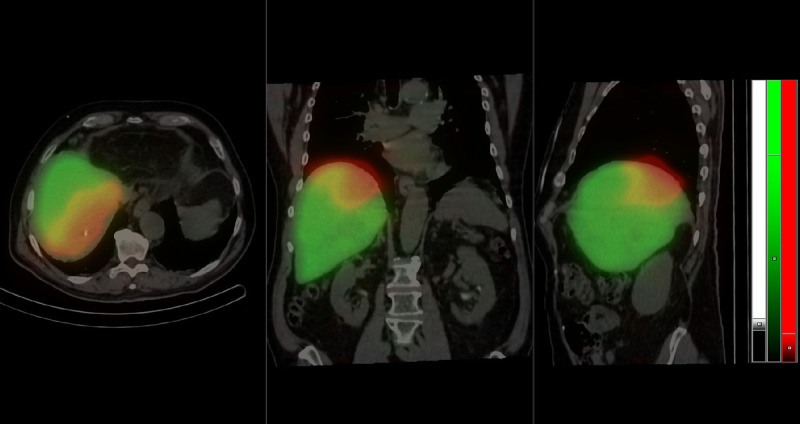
Fused ^99m^Tc-mebrofenin (green) and ^166^Ho (red) dual-isotope SPECT and CT images, transversal left panel, coronal middle panel, and sagittal right panel. Images were acquired on a Mediso SCP SPECT/PET/CT triple-modality camera (Mediso Medical Imaging Systems Ltd) equipped with a triple-detector SPECT after the transarterial injection of 140 MBq ^166^Ho-microspheres (QuiremScout; Quirem Medical B.V.) in the angiographic laboratory and the subsequent IV administration of 270 MBq of ^99m^Tc-mebrofenin. The healthy liver tissue included in the potential irradiation target of the planned radioembolization is marked by the yellow penumbra on the SPECT/CT images. The majority of the liver, however, expresses only ^99m^Tc-mebrofenin uptake (green), and the target encompassing the tumor is covered by the deposition of ^166^Ho-microspheres (red). Volumes of interests were drawn around the respective isotope distributions, and calculations from the encompassed volumes showed an estimated functional liver remnant (%FRL-C) of 85.5%, which was critically important considering that the patient had previously undergone a left hemihepatectomy. Fifteen days after the scout imaging, the radioembolization treatment was performed with 5 GBq of ^166^Ho-microspheres (QuiremSpheres; Quirem Medical B.V.) injected transarterially from the same catheter position. Posttreatment ^166^Ho-SPECT/CT images showed an identical microsphere deposition to that of the scout image set, and dosimetric evaluation showed an estimated mean absorbed dose of 37 Gy in the healthy liver and of 199 Gy in the tumor. Hepatic radioembolization with ^166^Ho-labeled polylactate acid spheres allows the pretreatment scout performed with identical spheres as to the treatment particles and the images to be acquired by SPECT, using the gamma emission of ^166^Ho (6.6% abundance of 81 keV photons).^1,2^ The intrahepatic distribution of ^99m^Tc-mebrofenin identifies healthy liver tissue, and it has been used for volumetric measurements before liver surgery to calculate total and future remnant liver volume^3–7^ and it is increasingly used in the workup of radioembolization procedures.^8,9^ The feasibility of dual-isotope acquisition of ^99m^Tc-colloid and ^166^Ho-microspheres has been shown^10,11^; however, we suggest that hepatocyte-specific mebrofenin is more suitable for future liver remnant investigations in the dual-isotope imaging setting than labeled colloids that are proportional to the activity of the reticuloendothelial system.^12,13^ Here, we presented a case underlining that dual-isotope acquisition of ^99m^Tc-mebrofenin and ^166^Ho is clinically feasible and the complementary information gained from this examination helps to plan radioembolization strategies where future remnant liver function is equivocal. This dual-isotope SPECT/CT acquisition has the advantage of imaging the patient in the same position and that only one low-dose CT scan is necessary for attenuation-correction compared with the setting where ^99m^Tc-mebrofenin functional volumetry and ^166^Ho-scout SPECT/CT is performed at different time points.
